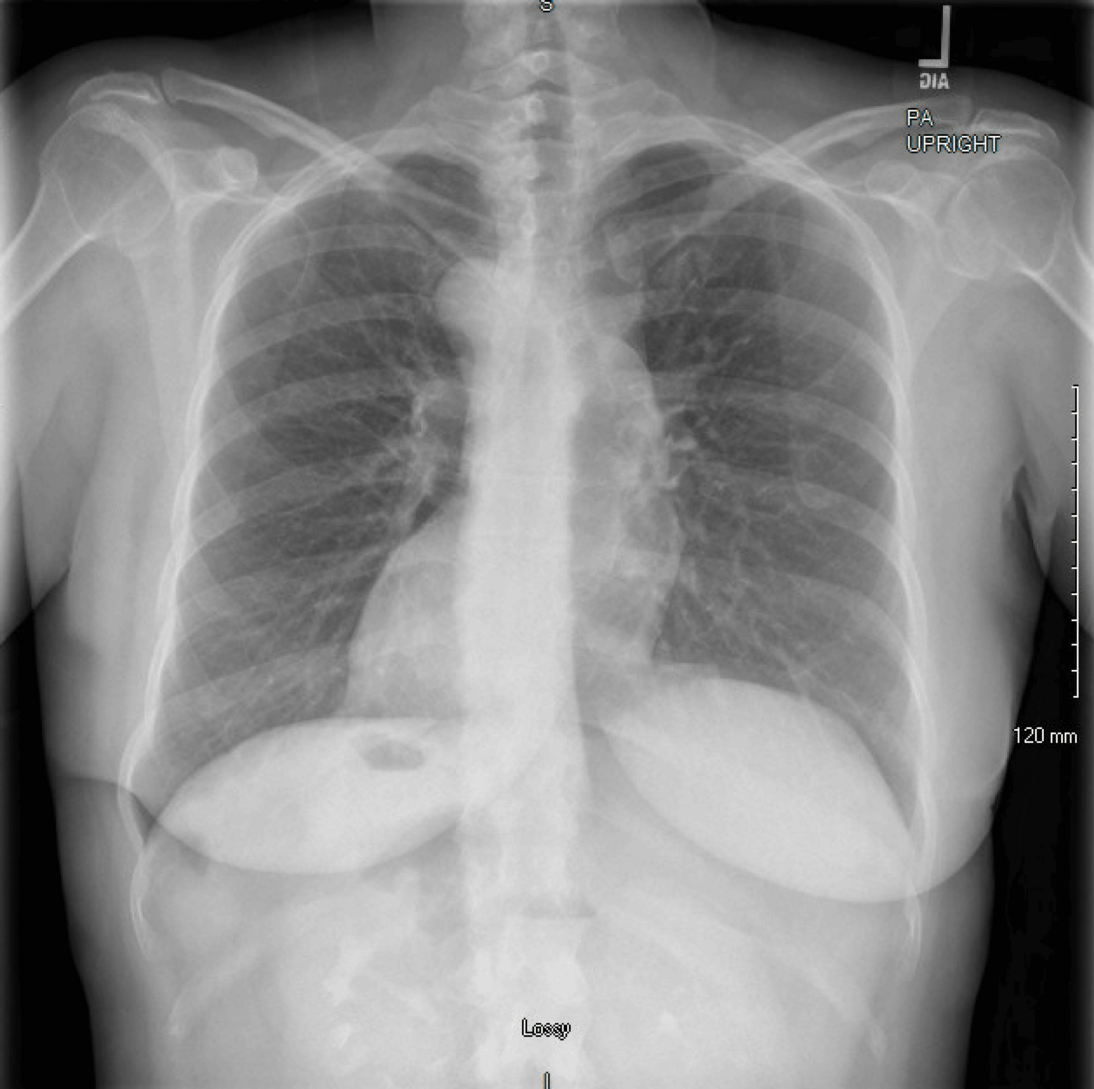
Chest X-ray demonstrating dextrocardia consistent with SIT. Note: The gastric bubble is located in the right upper quadrant of the abdomen as opposed to the left upper quadrant SIT: situs inversus totalis
